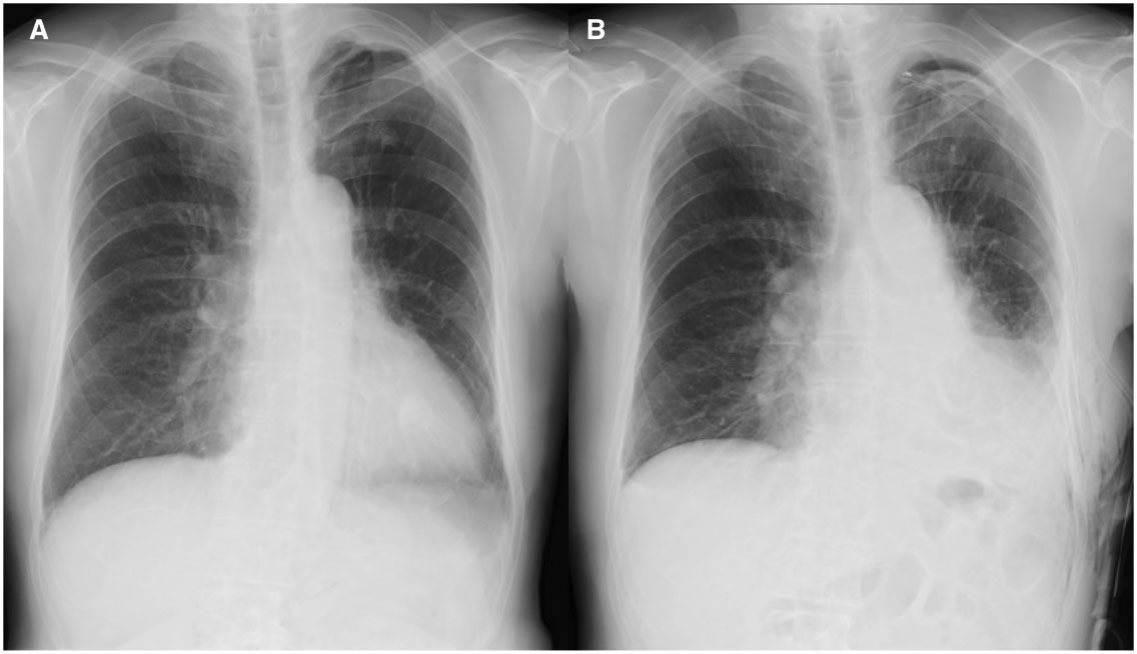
Pre- and postoperative chest radiographs. (A) The preoperative chest radiograph showed a leftward shift of the heart and a dull cardiac diaphragm. The trachea remained in the midline. (B) The postoperative chest radiograph showed no cardiac or mediastinal deviation.
